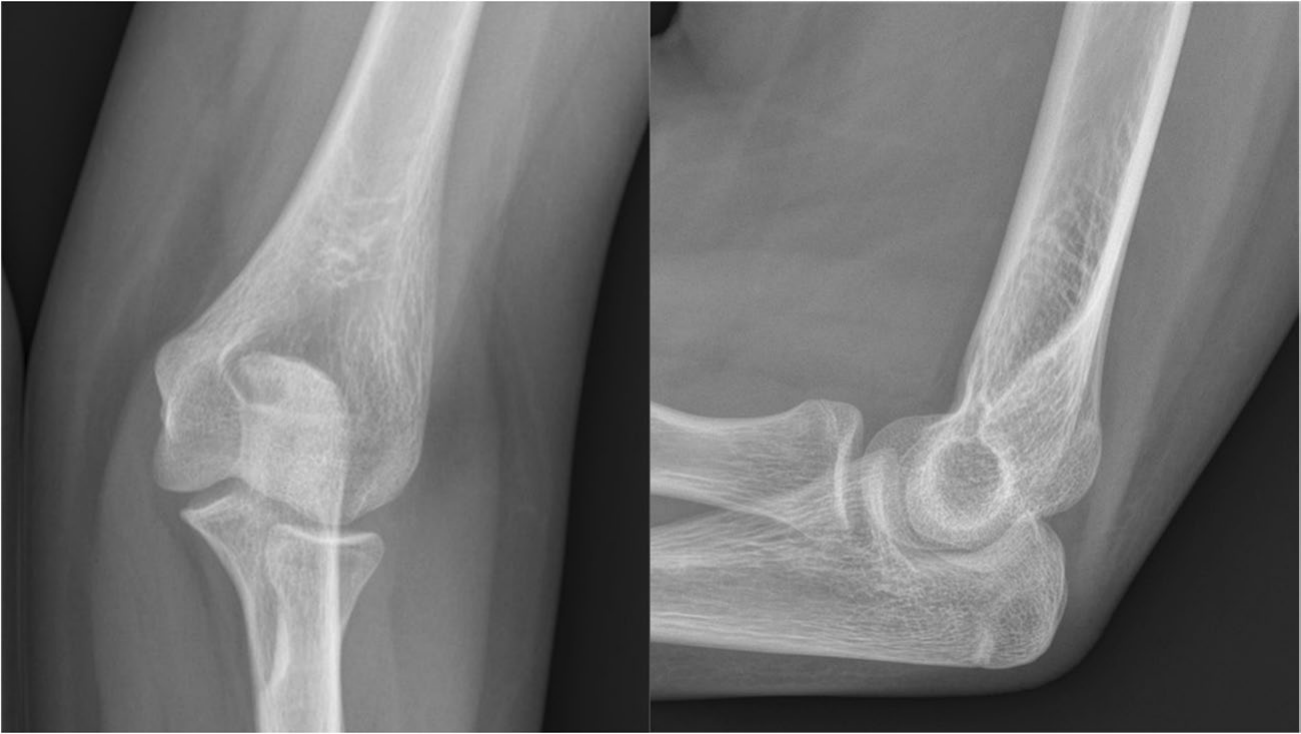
AP and L view radiograph of the elbow: final result, four years after surgical treatment
